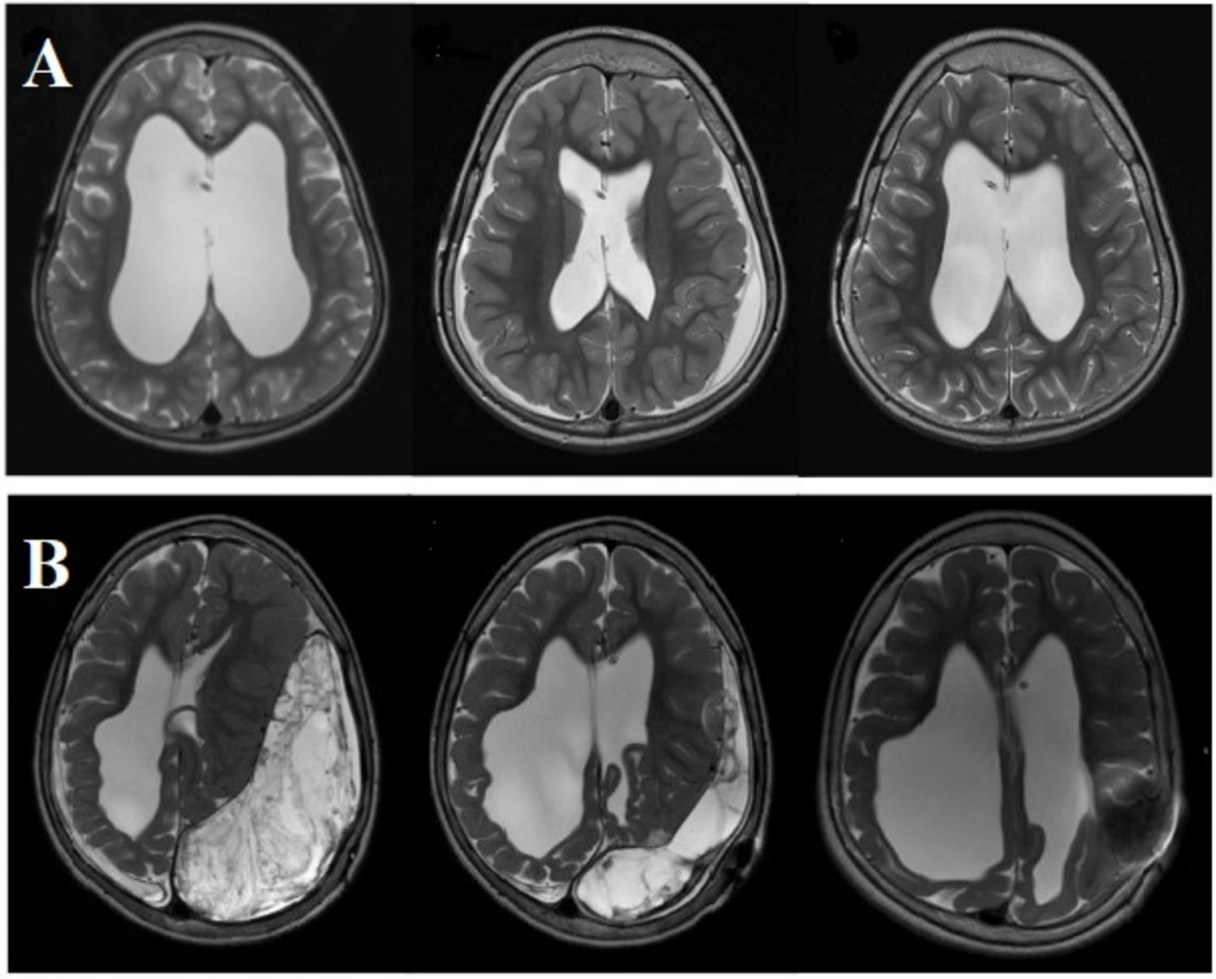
Follow-up MRI scans of two different pediatric patients with hydrocephalus who were surgically treated with VPs. Both patients presented with clinical and radiological signs of overdrainage. A Showed hyperostosis and subdural hematoma, while (B) exhibited a subdural hygroma. Both cases were successfully managed through shunt pressure level adjustments
